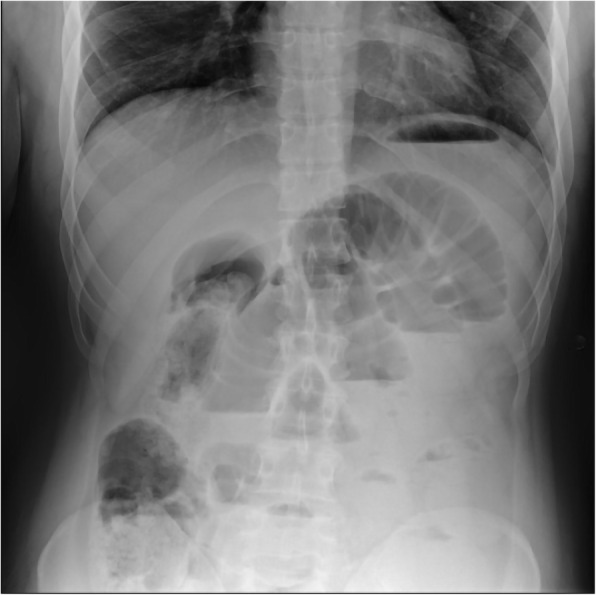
Abdominal X-rays showing small bowel distension with air-fluid levels
